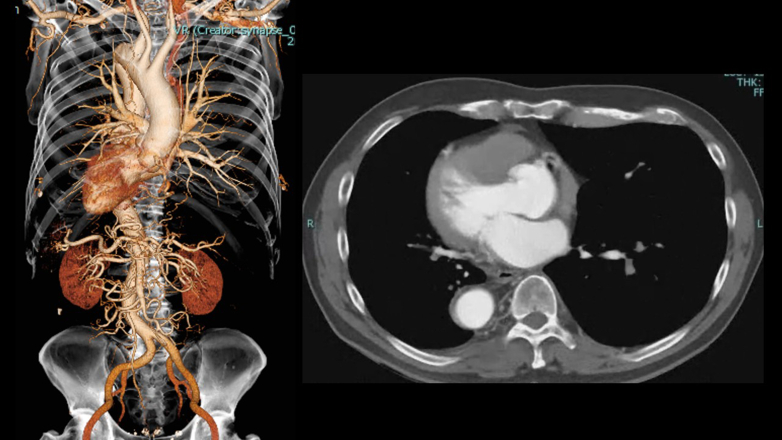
Situs inversus totalis confirmed by 3-dimensional reconstruction and axial computed tomography images showing mirror-image positioning of thoracoabdominal organs.
